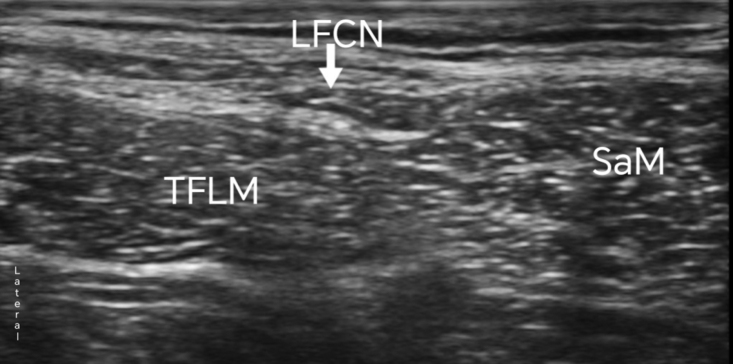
Ultrasound image of lateral femoral cutaneous nerve block.
